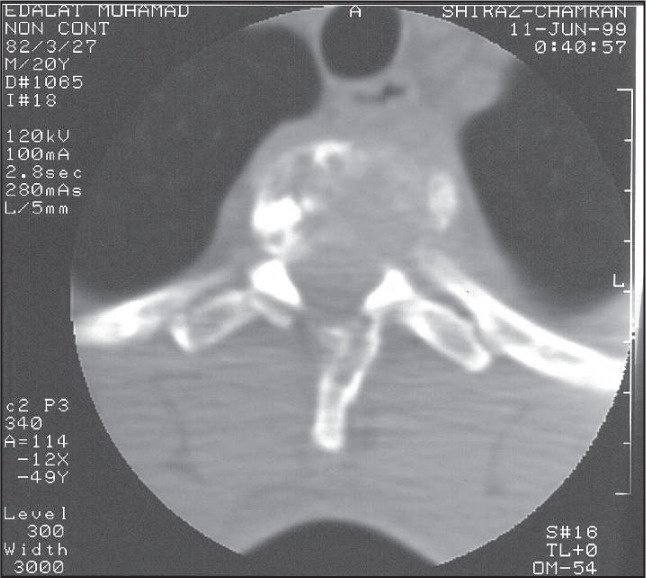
Axial computed tomography scan of the chest showing the expansion and destruction of the T2 vertebral body, with soft tissue extending to the spinal canal in a young man with hemangiopericytoma.
